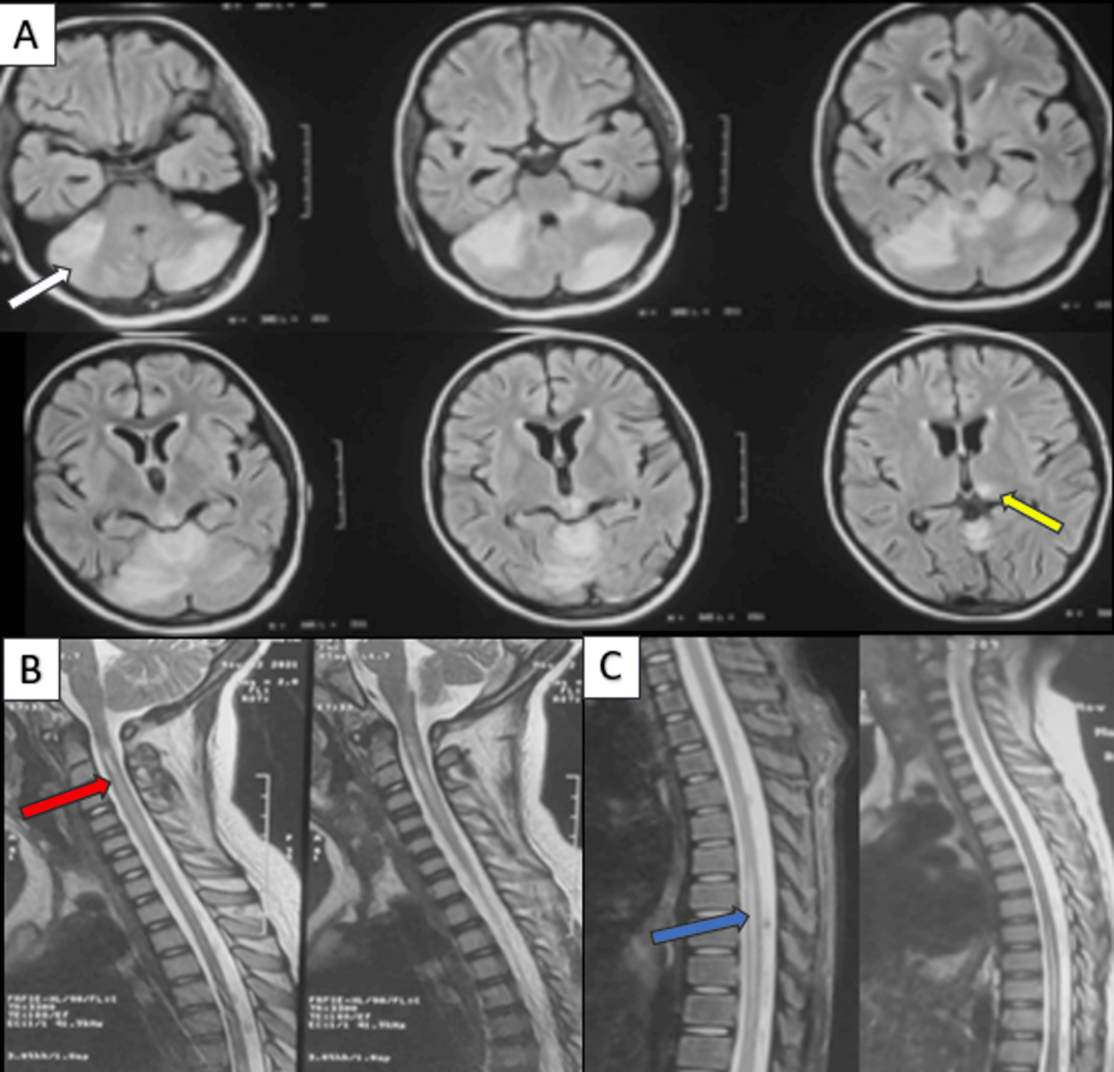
MRI axial FLAIR brain (A), sagittal T2W (B) cervical spine, and sagittal T2W (C) dorsal spine images. An 11-year-old female, a case of systemic lupus erythematosus, presented with headache, visual blurring, and swaying while walking. Her initial blood pressure recordings were high. Fundoscopy was suggestive of hypertensive retinopathy. She was treated with pulse steroids and antihypertensives and showed significant clinical improvement on day three. Brain MRI on day two of presentation revealed FLAIR (A) hyperintensities in both cerebellar hemispheres (white arrow) and left thalamus (yellow arrow). Cervical (B) and dorsal (C) spine MRI revealed central T2 hyperintensities in the cervical (red arrow) and thoracic (blue arrow) spinal cord. Follow-up brain imaging on day seven revealed complete resolution. Spine imaging after four weeks revealed significant resolution. T2W: T2-weighted; FLAIR: fluid-attenuated inversion recovery.
